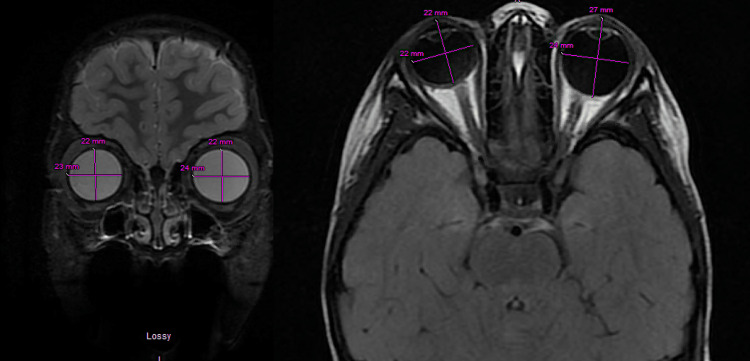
Magnetic resonance imaging of the Brain T1 FLAIR from 2023 showing asymmetric axial elongation to 27 mm of the left eye, compared to 22 mm in the right eye.
